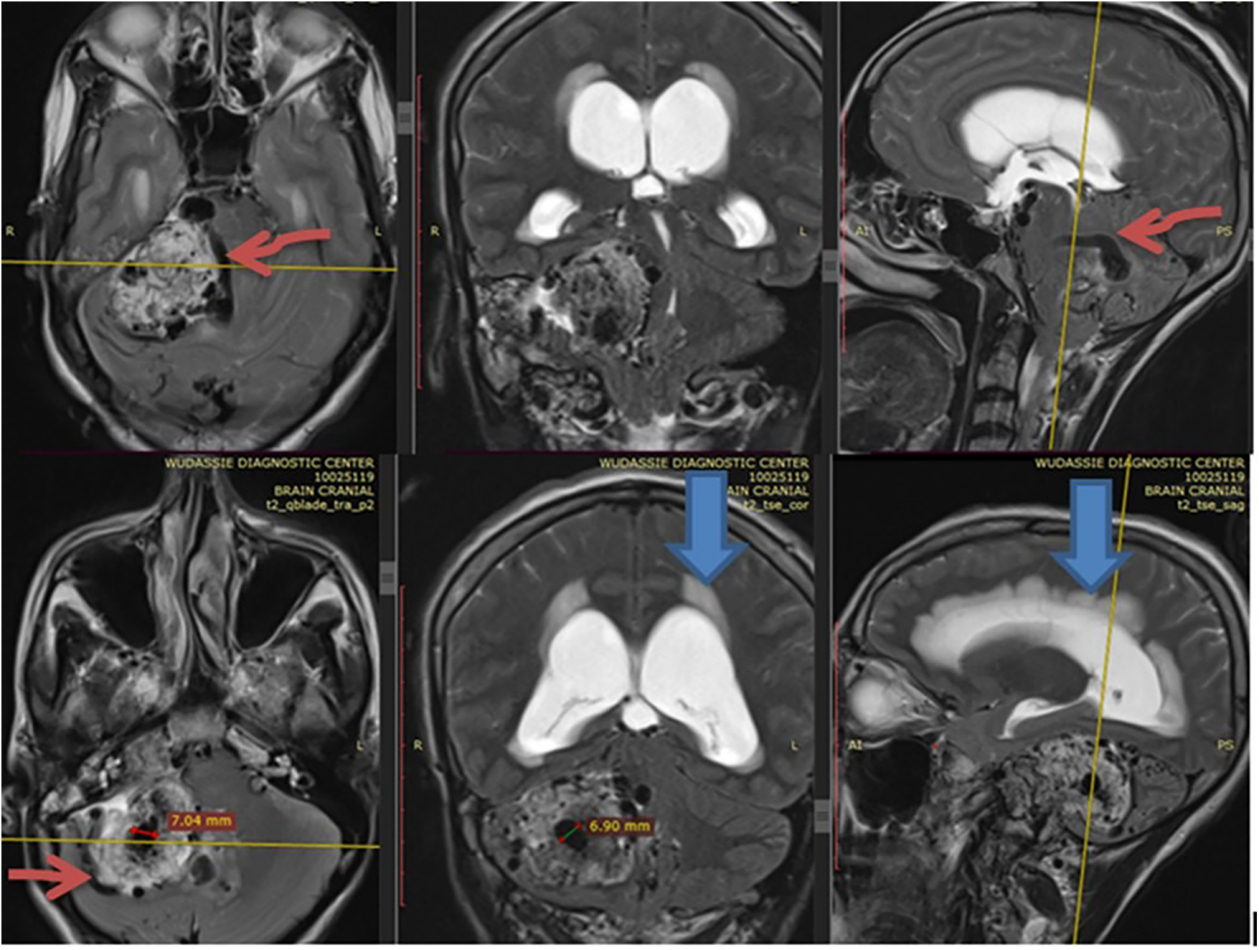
Shows with extensive multiple & large size intra & peritumoral flow voids (depicted by the dark red) as it appears hypointense on T2 MRI in axial, coronal and sagittal section. The blue arrow also shows acute hydrocephalus.
